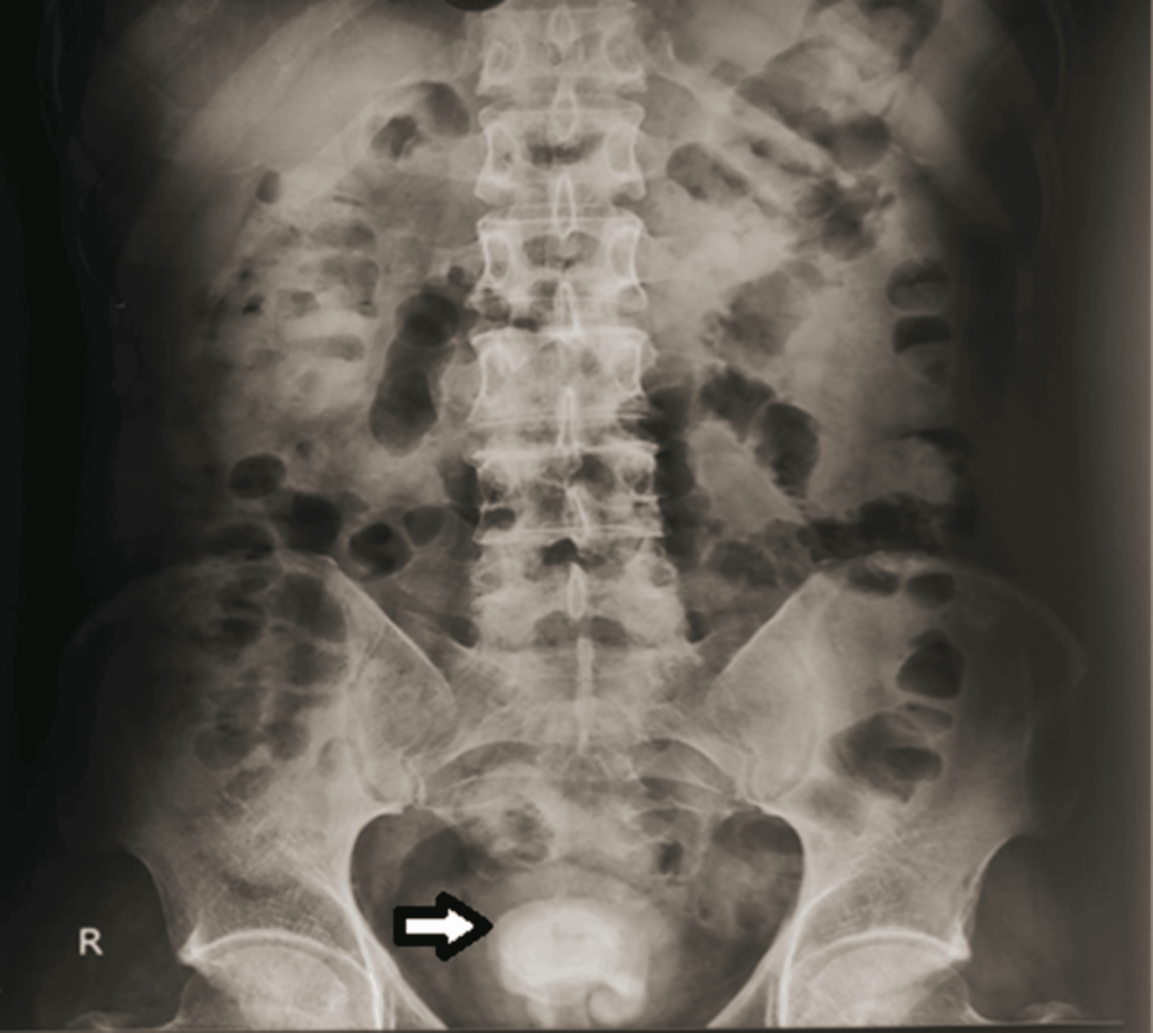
X-ray KUB showing radio-opacity in the bladder region (arrow) KUB: Kidney ureter bladder
